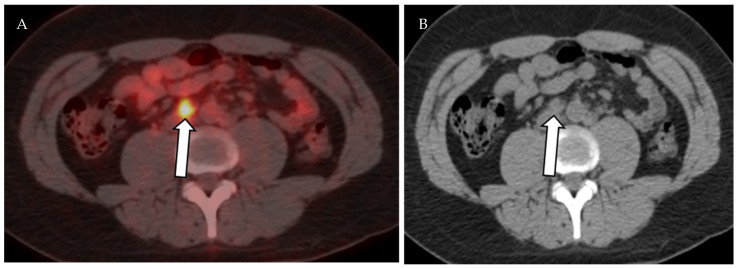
Axial fused PET-CT (A) and non-contrast CT of the abdomen shows mildly enlarged right paraaortic lymph node with increased FDG avidity on PET-CT (SUVmax 5.7), (B) biopsy proven Hodgkin lymphoma.
